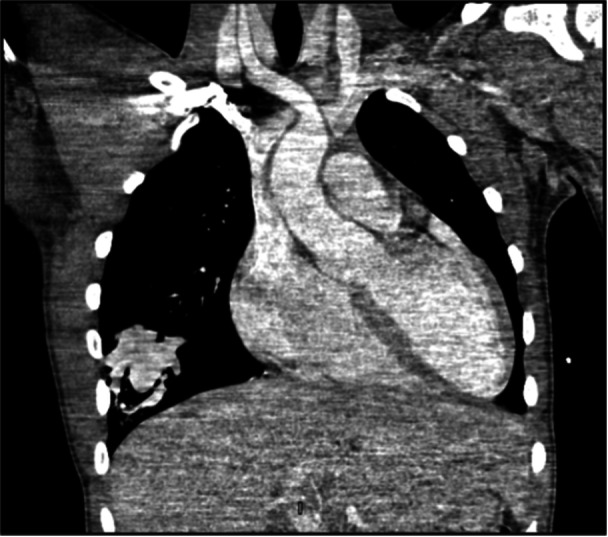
Computed tomography (CT) angiography confirming pulmonary AVM. CT angiography (CTA) revealing a pulmonary arteriovenous malformation (AVM) in the right lower lobe, characterized by a dilated feeding artery and early venous return, explaining the patient′s positional hypoxia.
